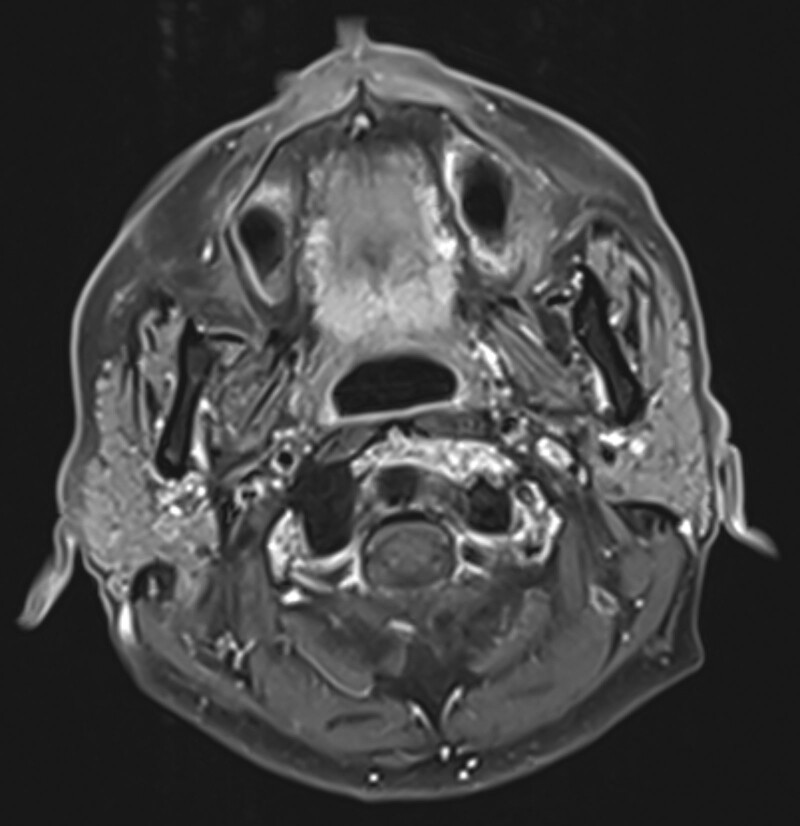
MRI showed a solitary enhancing lesion with normal marrow replacement and cortical bone breakthrough in the left anterior arch of the C1 vertebra. MRI = magnetic resonance imaging.
